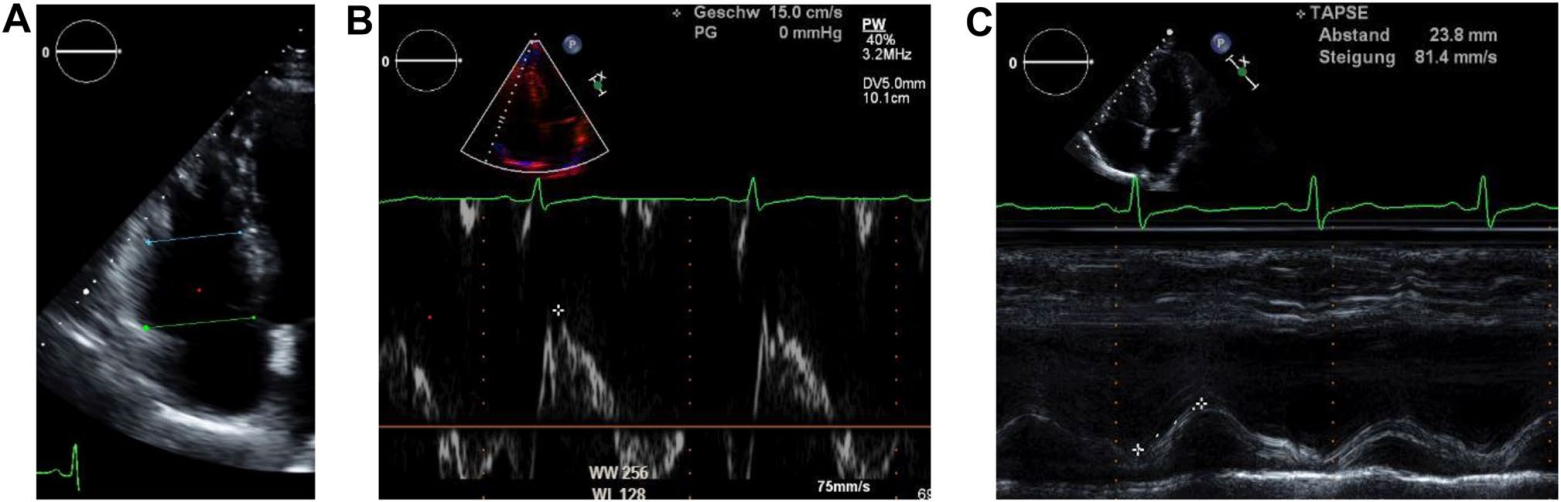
A RV diameter at base (green line): linear dimension measured end-diastolic at the basal one third of the RV; RV diameter mid-level (blue line): linear dimension measured end-diastolic in the middle third of the RV; B representative example of TAPSE (a parameter of RV longitudinal function and is measured from the tricuspid lateral annulus in M-Mode); C representative example of TDI S’ (the systolic velocity of lateral tricuspid annulus by pulsed tissue Doppler)
